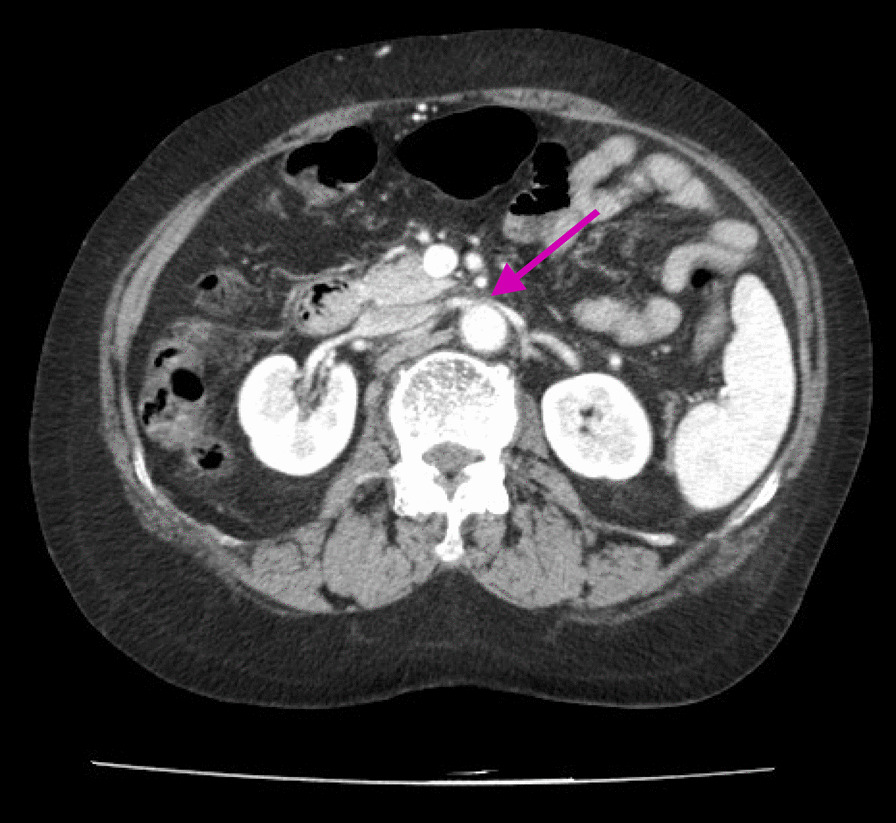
Computed tomographic image shows severe stenosis of the left renal vein. The purple arrow indicates the beak sign
